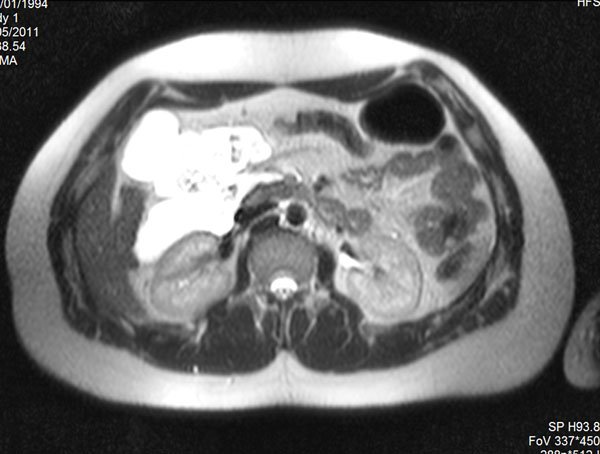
MRI of the abdomen. Fluid - filled mass (18x12 cm) with internal septa located between the stomach and the pancreas with lateral extension in the hepatorenal space.
